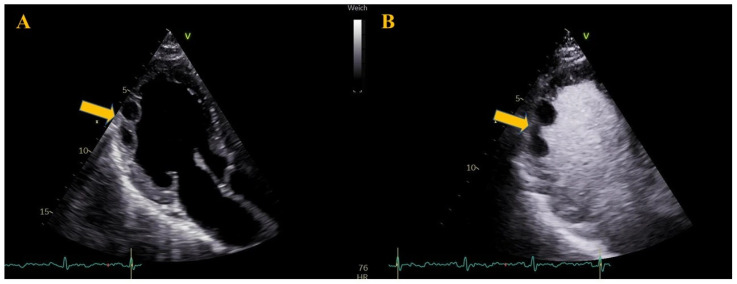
Day 6. Transthoracic echocardiography, apical three-chamber view. Panel (A): Thrombus progression despite triple-therapy, now appearing as two mobile masses (14 × 12 mm and 18 × 11 mm) attached to the mid-to-apical anterolateral wall. Panel (B): Contrast-enhanced left heart echocardiography demonstrating improved thrombus delineation.
